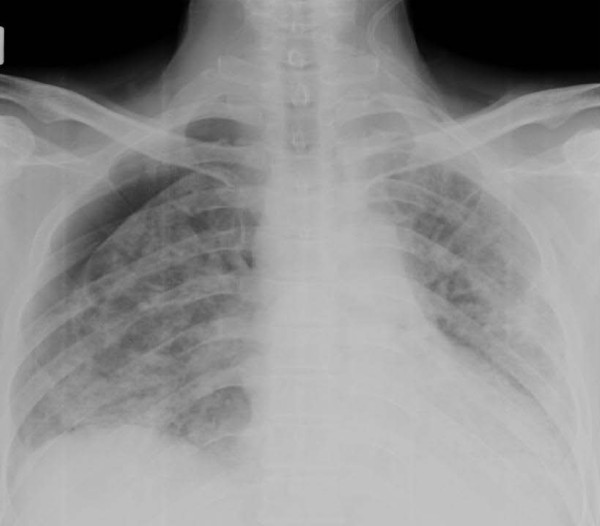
Chest radiography reveals pneumothorax of right lung.
